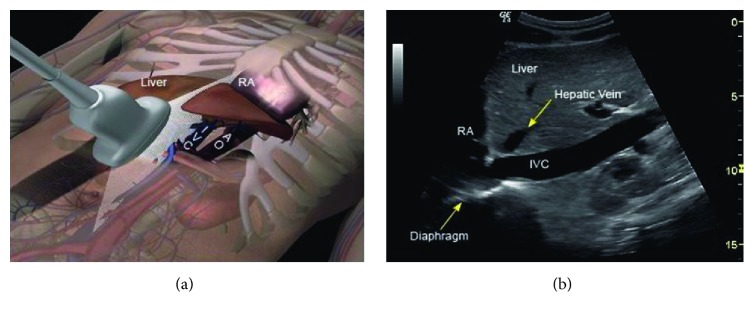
Subcostal inferior vena cava landmarks. (a) Position of ultrasound probe for visualization of the inferior vena cava (IVC) (see Figure 1, probe position B1). The IVC is located to the right of midline and aorta (AO). (b) Corresponding ultrasound image of the IVC. The IVC is typically measured 2 cm from the right atrium (RA) or just distal to the hepatic vein. The hepatic vein junction to IVC and the IVC junction to right atrium are confirmatory landmarks. Reproduced with permission from Killu et al. [26].
